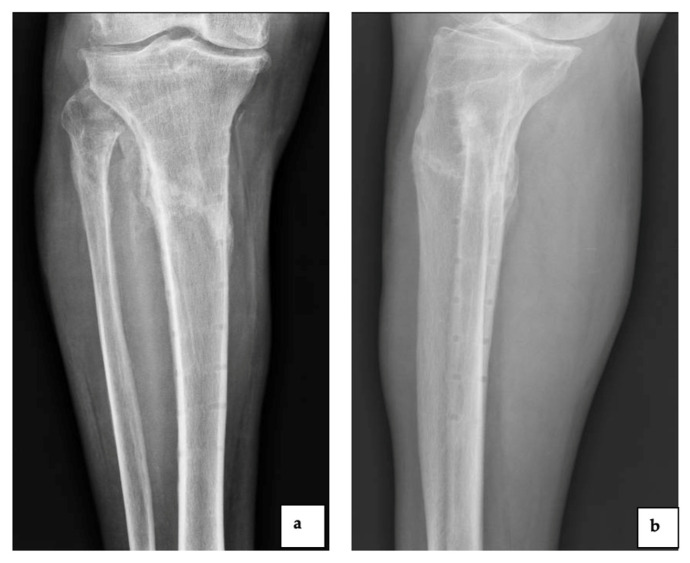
The plain radiograph after removal of the tibial plate at 6 months of follow-up ((a): anteroposterior view and (b): lateral view).
